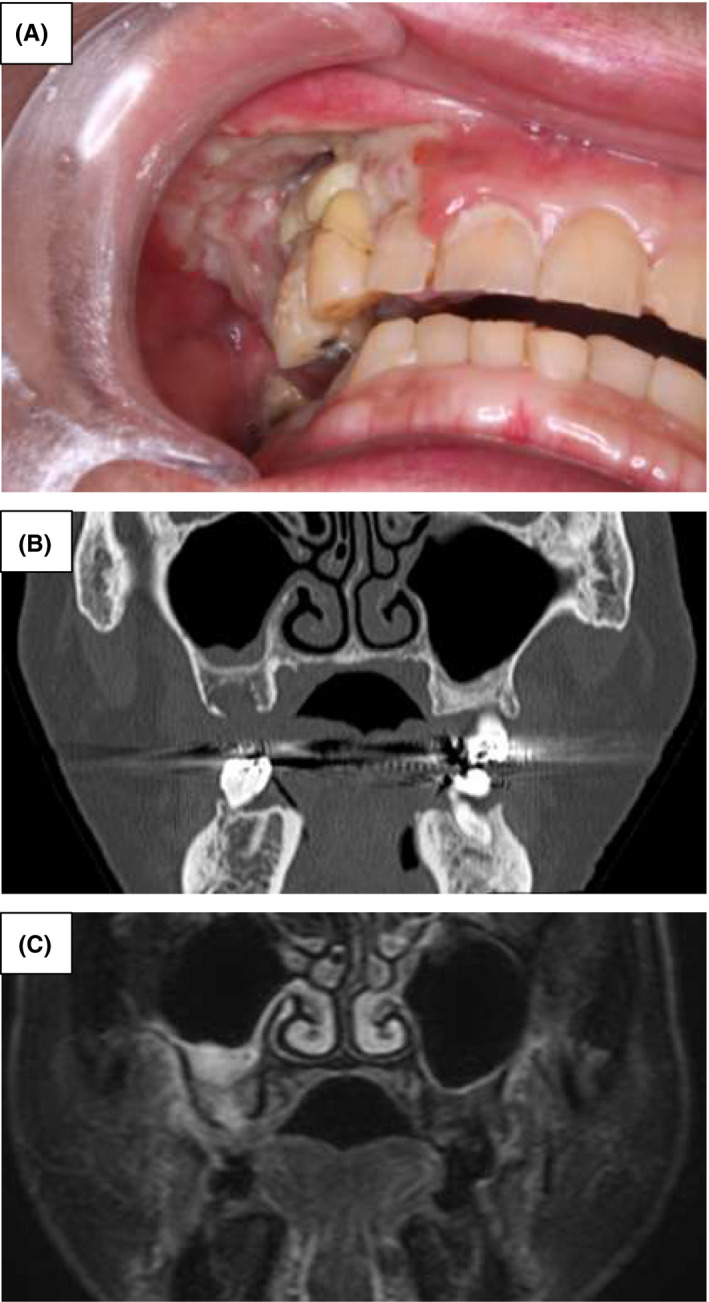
Oral cavity at the initial examination. A, Large ulceration with no peripheral induration, infection with purulent discharge, and exposed bone in the ulcer were noted on the right maxillary gingiva. B and C, Computed tomography and magnetic resonance imaging showed osteosclerosis in the right maxillary jaw bone, and the upper right maxillary sinus mucosa was hypertrophic
